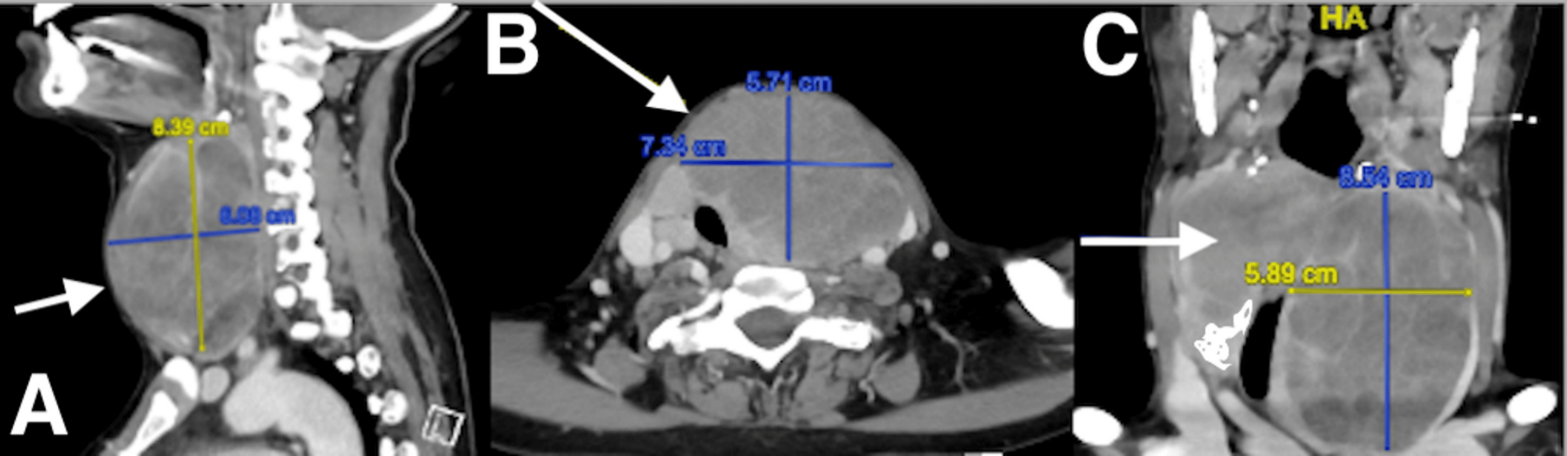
First computed tomography (CT) of the thyroid (A) Sagittal, (B) transverse horizontal, and (C) coronal dimensions on computed tomography (CT) of the anatomical thyroid bed pre-total thyroidectomy (arrows).
